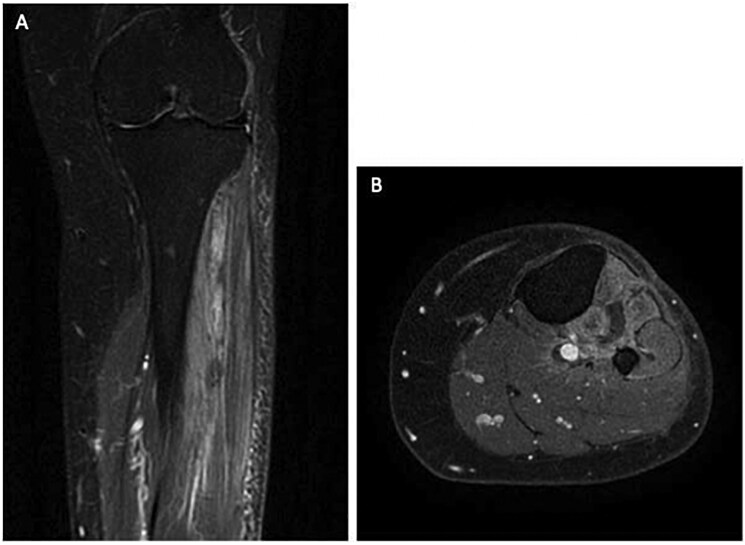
Post-surgical MRI of residual myxoid liposarcoma. (A) Coronal T1 fat-saturated post-contrast image; (B) Axial T1 fat-saturated post-contrast image. Minimal residual neoplastic tissue is visible as irregular contrast enhancement in the interfascial area.
